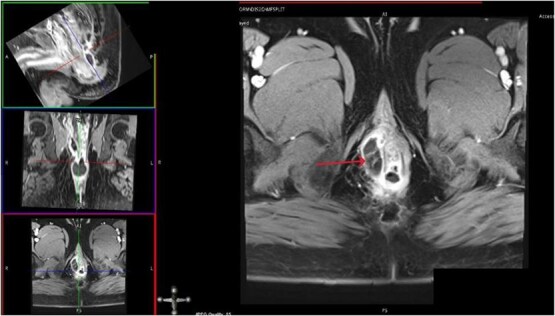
MRI of the anus revealing the septae within the abscess.
